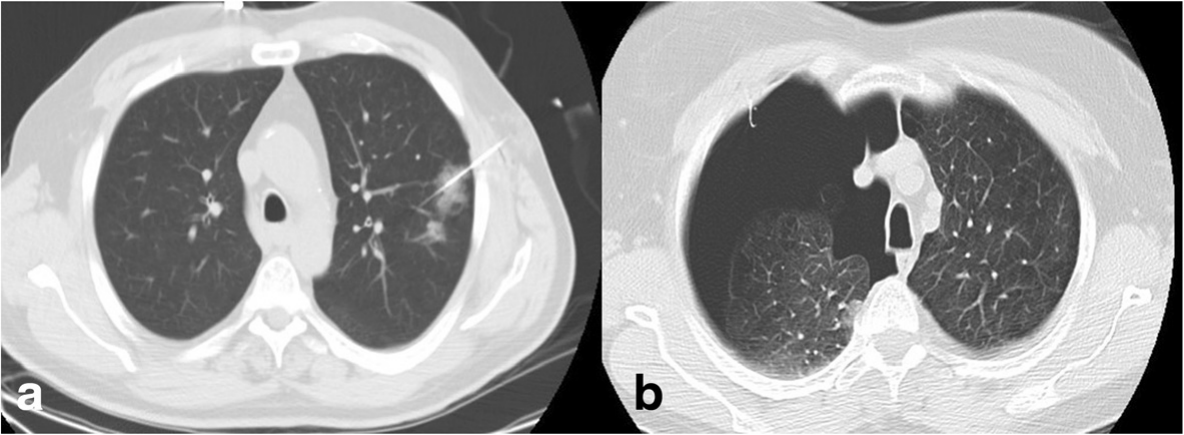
a Axial CT-image shows parenchymal bleeding of the left upper lobe located around the needle of the hook wire after insertion. b Axial CT-image demonstrates an important pneumothorax of the right lung occurring after hook wire insertion requiring chest tube drainage
